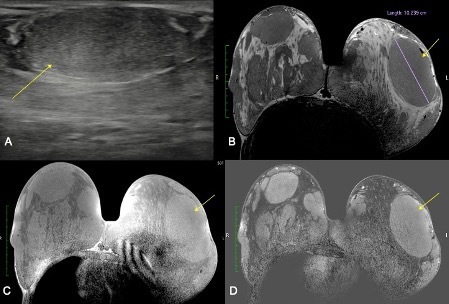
Breast ultrasound (A) and MRI in *T_2_
